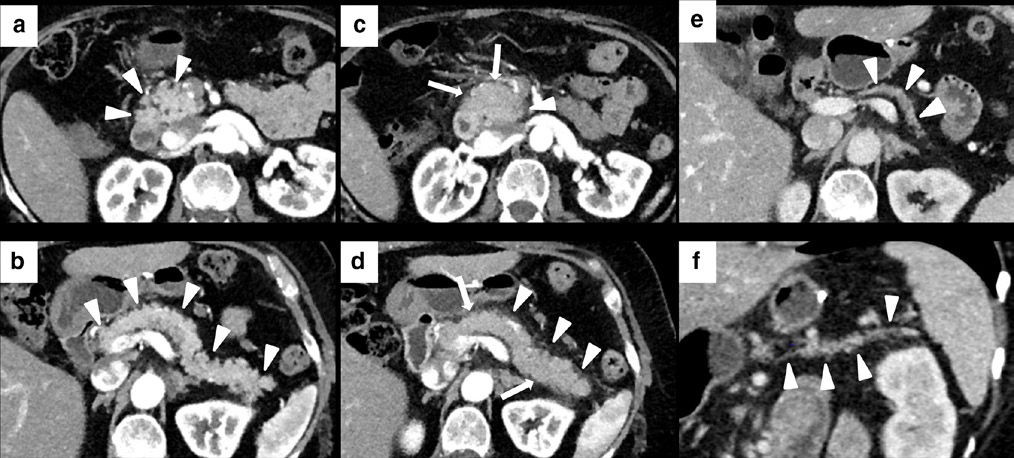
Imaging of ICIPI in a 61-year-old female with metastatic melanoma, treated with nivolumab. Baseline CECT showed normal appearance of the head (white arrowheads in A) and the body-tail (white arrowheads in B) of the pancreas. At follow-up after 4 months, patient presented dyspepsia, epigastric pain, diarrhea and elevation of lipase and amylase blood level. CT images showed a slight and homogeneous enlargement of the whole pancreas with loss of the physiologic lobulation (white arrows in C and D) associated with a subtle peripancreatic fat stranding (white arrowheads in C and D). This pattern was classified as IEP. ICI therapy was discontinued until normalization of blood lipase and amylase levels. Follow-up CT showed post-pancreatitis parenchymal atrophy (white arrowheads on axial and coronal CT, respectively, in E and F).
